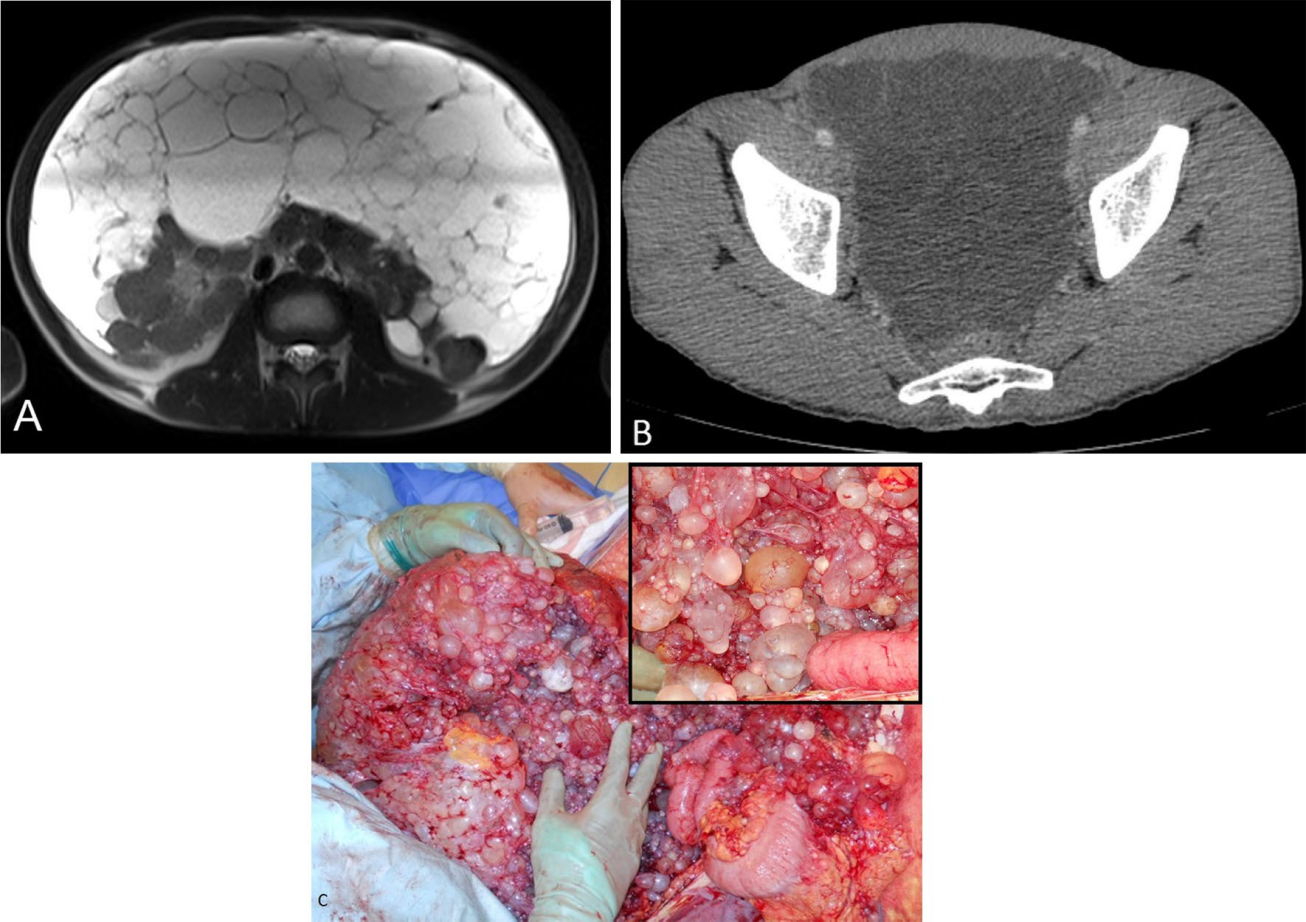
a–c 42-year-old male with diffuse form of cystic mesothelioma. a MRI T2 weighted axial image shows diffuse multi-cystic lesions in the peritoneal cavity. b CT through the pelvis shows fluid attenuation cystic lesions with fine non-enhancing septations. c Intra-operative photograph shows diffuse multi-loculated cystic masses in the diffusely filling the peritoneal cavity
